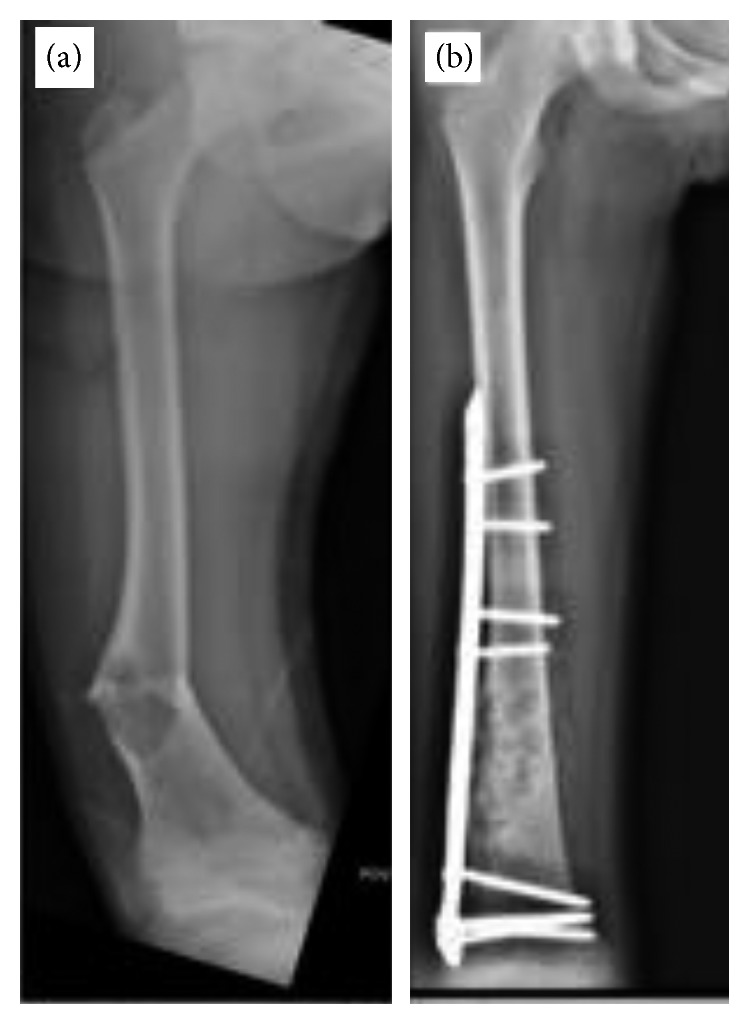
(a) AP X-ray with pathological fracture through a diaphyseal ABC. (b) AP X-ray after intralesional resection/curettage followed by fracture fixation with plate and screws.
